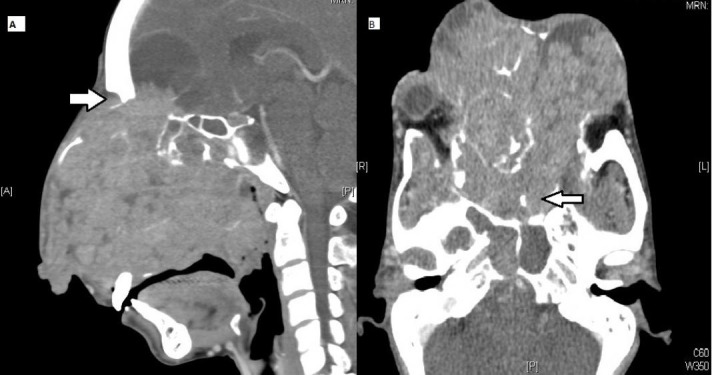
Pre-treatment CT (A) Sagittal section showing a large exophytic tumor with breach of the anterior cranial fossa (white arrow) and involvement of the sphenoid sinus. (B) Axial section demonstrating displacement of the orbit and invasion of the nasopharynx (white arrow). CT: Computed tomography.
